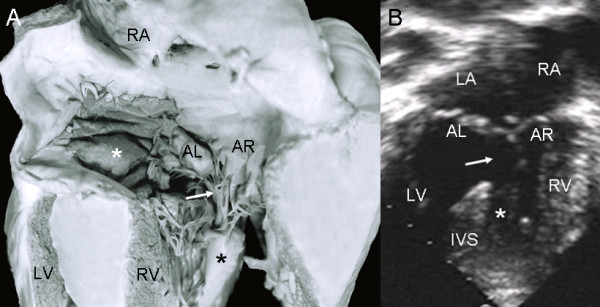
Atrioventricular septal defect with common valve with Rastelli type B insertion. (A) View of right heart chambers. Surgical patch is visible over atrioventricular septal defect (white asterisk) and the insertion of anterior leaflets to a papillary muscle (black asterisk) joined to the apical portion of the ventricular septum. (B) The two-dimensional echocardiographic 4-chamber image shows a similar insertion. Abbreviations as before.
